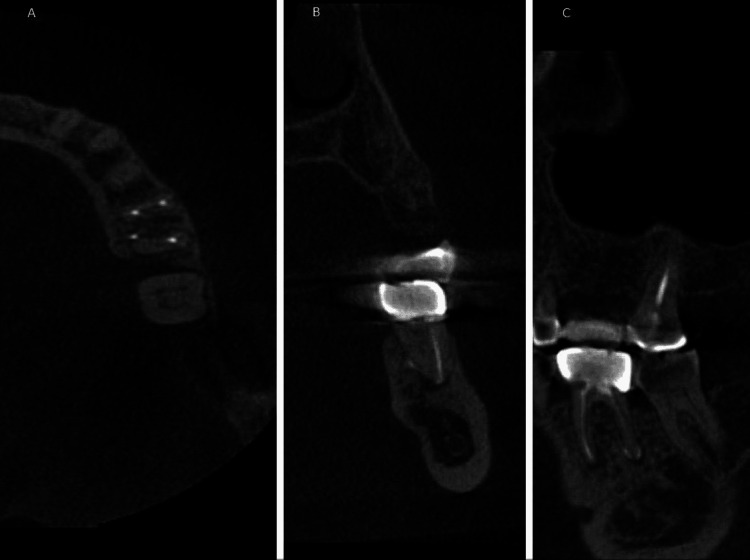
Cone-beam computed tomography of molar #36 with apical radiolucency on distolingual root because of the untreated distolingual canal. A: The axial plane; B: the coronal plane; C: the sagittal plane.
